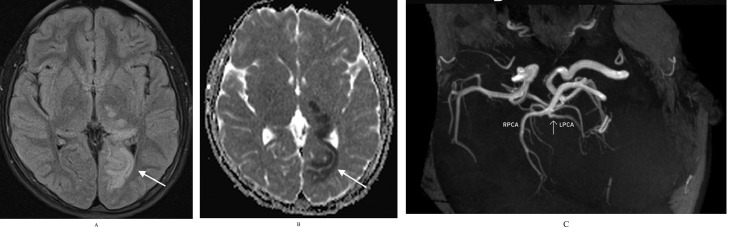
MRI on Day 1. (A) There exists a high signal in the cortex of the inner surface of the left occipital lobe and in the left thalamus (arrow). (B) The lesions have a low signal on the ADC map characteristic for ischemia. C) On a 3D-TOF oblique projection, there is a narrowing at the P1-P2 junction while the P4 segment is not apparent.
